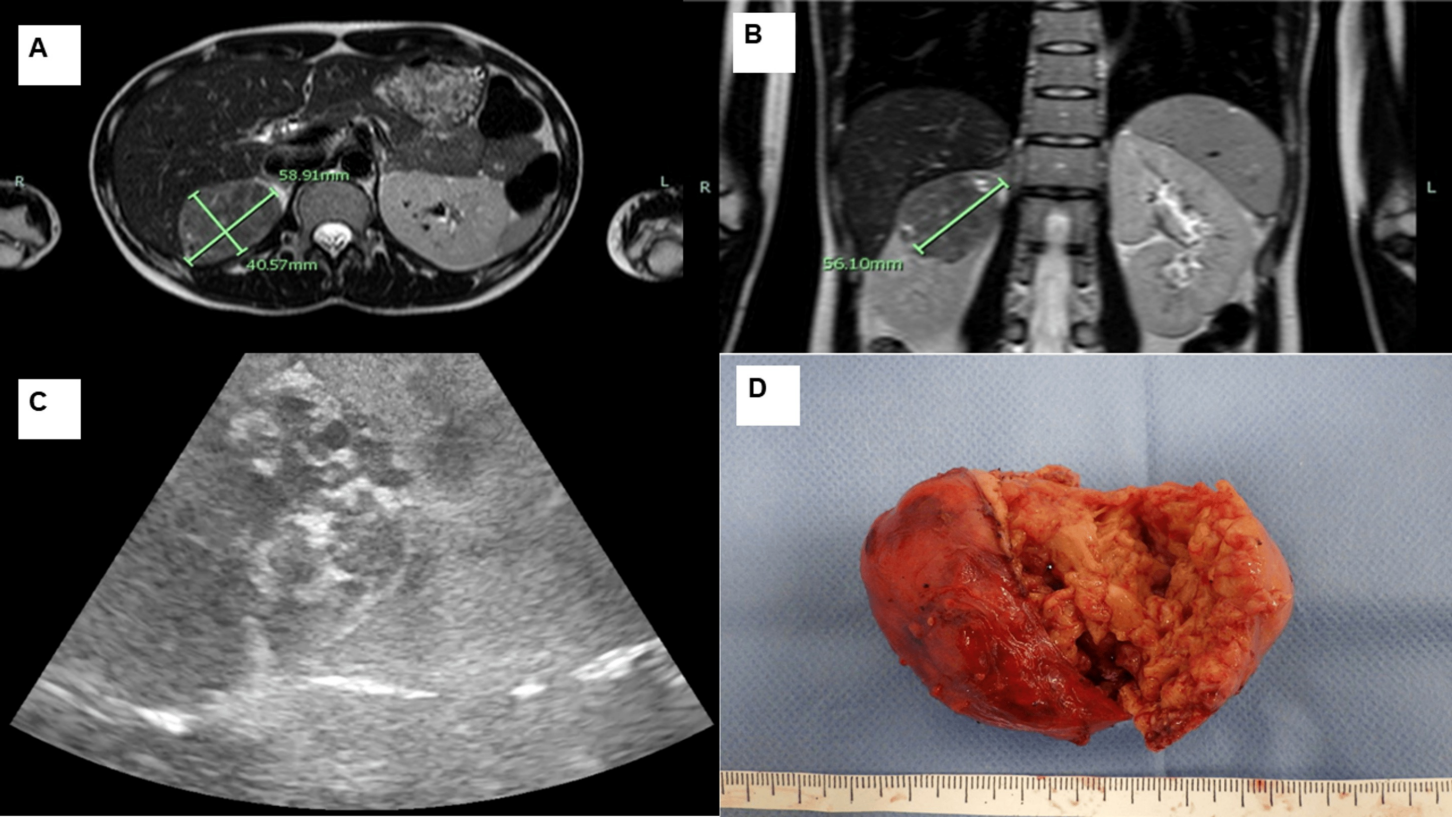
Magnetic resonance imaging (MRI), intraoperative echography, and gross image of the tumor Magnetic resonance imaging showing a 5.8 × 4.0 × 5.6 cm tumor originating from the upper pole of the right kidney. A: T2-weighted imaging (WI), transverse section; B: T2WI, coronal section; C: intraoperative echogram showing a well-defined borderline mass; D: Resected right kidney tumor with the size of 41 x 58 x 49 mm.
